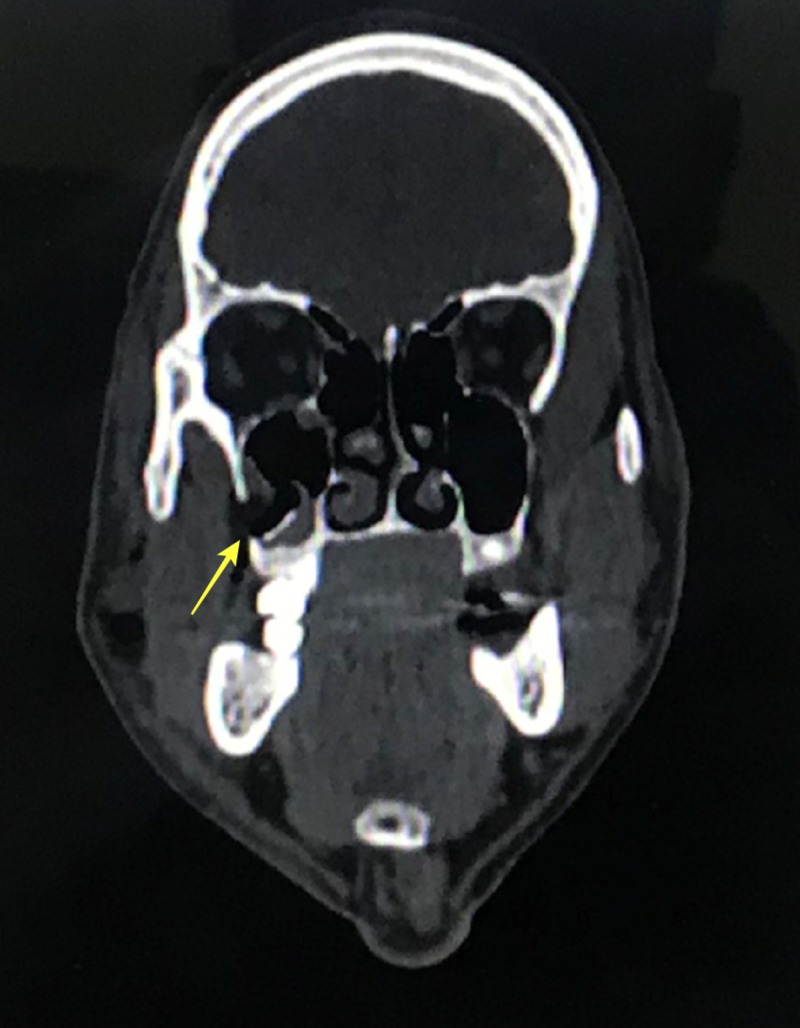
Coronal computed tomography view demonstrating fracture in the right zygomatic maxillary buttress region
